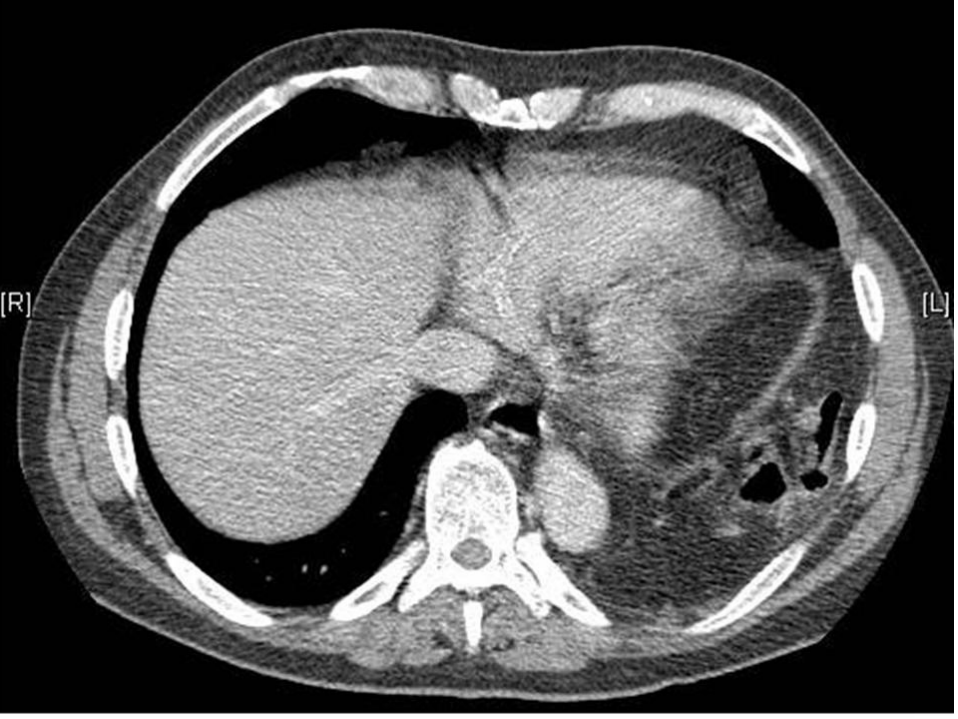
Axial post IV contrast CT through the lower chest/upper abdomen showing loops of bowel herniating through the disrupted left hemidiaphragm.
